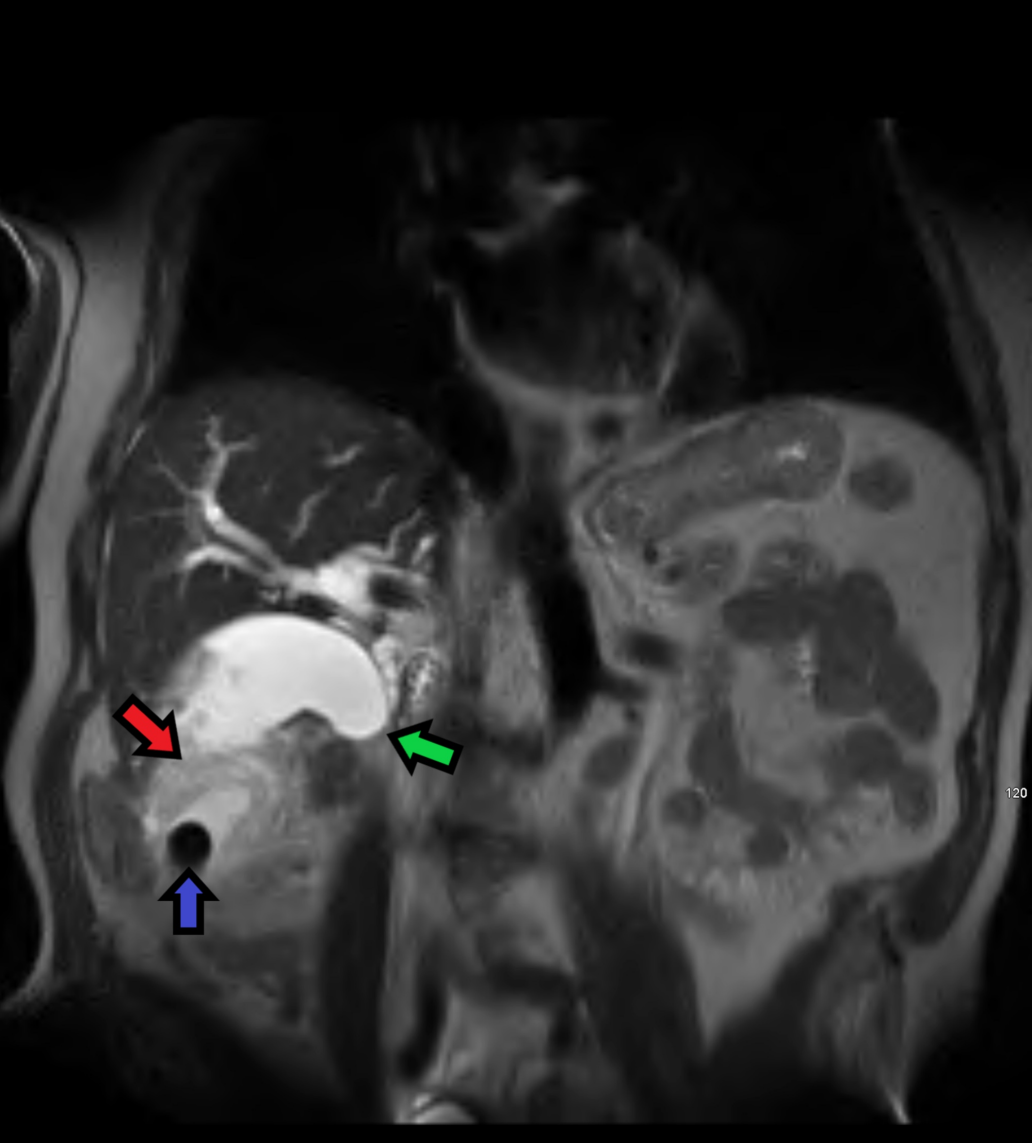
Coronal MRI T2 HASTE weighted image demonstrating a large mass (red arrow) surrounding a gallstone (blue arrow) in the distal portion of the gallbladder fundus. There is sharp tapering in the mid-to-distal common bile duct (green arrow) indicating obstruction secondary to infiltration by the mass MRI - Magnetic resonance image, HASTE - Half-Fourier Acquisition Single-shot Turbo Spin-Echo
